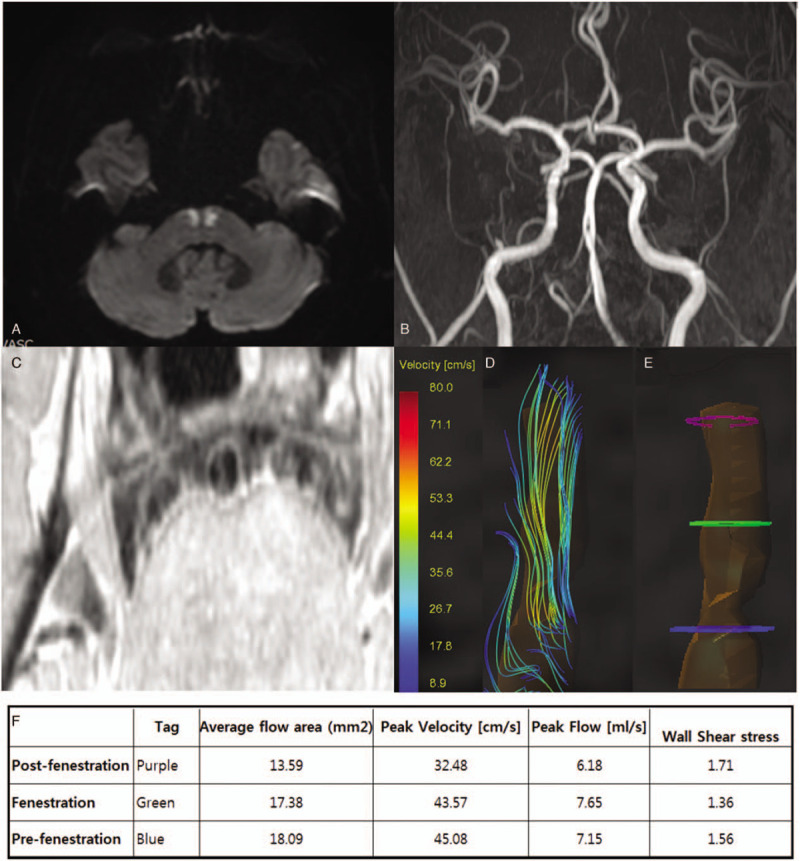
Bilateral pontine infarction with basilar artery fenestration. Axial diffusion-weighted image MRI (A) with symmetric bilateral pontine infarction sparing the midline of basis pontis and time-of-flight magnetic resonance angiography (B) show basilar artery fenestration. High-resolution MRI (C) reveal intraluminal wall and dual lumen in the basilar artery. Basilar artery flow trajectory presented at systolic (D) period demonstrating bifurcated blood flow. Hemodynamic parameters measured from pre-fenestration (blue), fenestration (green), and post-fenestration (purple) area (E) shows low wall shear stress at the fenestration area (F).
